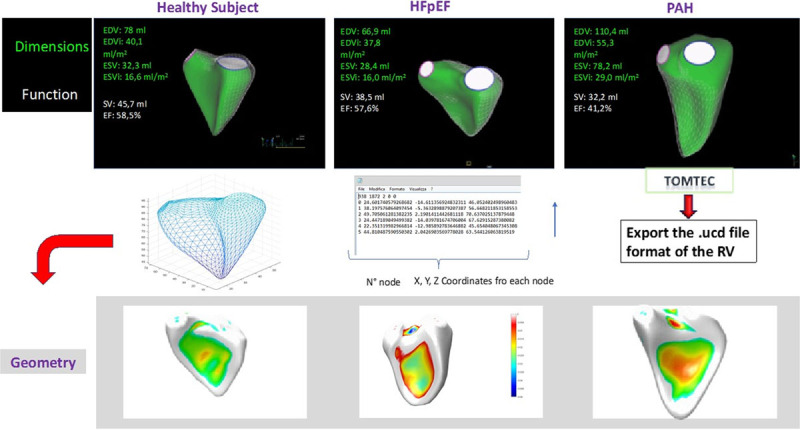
Three-dimensional right heart echocardiography reconstruction and analyses Dimensional, functional, and geometric data of a healthy subject (A) versus a heart failure with preserved ejection fraction (HFpEF; B) and a pulmonary arterial hypertension (PAH) patient (C). Postprocessing analysis of nodes and colorimetric scale reconstruction of the right ventricular (RV) shape intriguingly shows that the main element in the normal RV function is the midposition of the septum with early HFpEF compared with control already shows some bulging of the septum in the left ventricle which is actually typical of PAH condition. EDV indicates end-diastolic volume; EDVi, end-diastolic volume indexed; EDSi, end-systolic volume indexed; EF, ejection fraction; ESV, end-systolic volume; SV, stroke volume.
